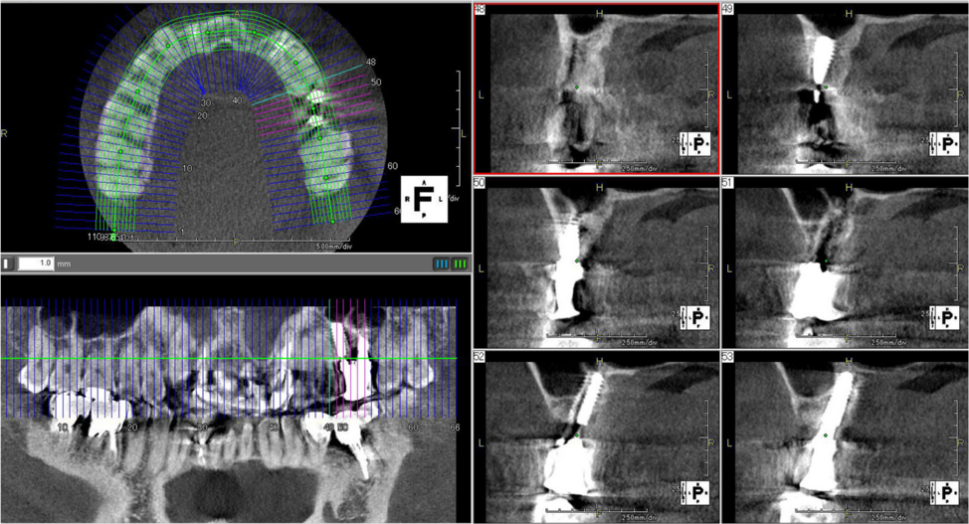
Case 2. Cone-beam CT scan obtained in February 2013 (4 years and 3 months after superstructure placement), showing that the double implants were surrounded by enough hard tissue to support the double implants
